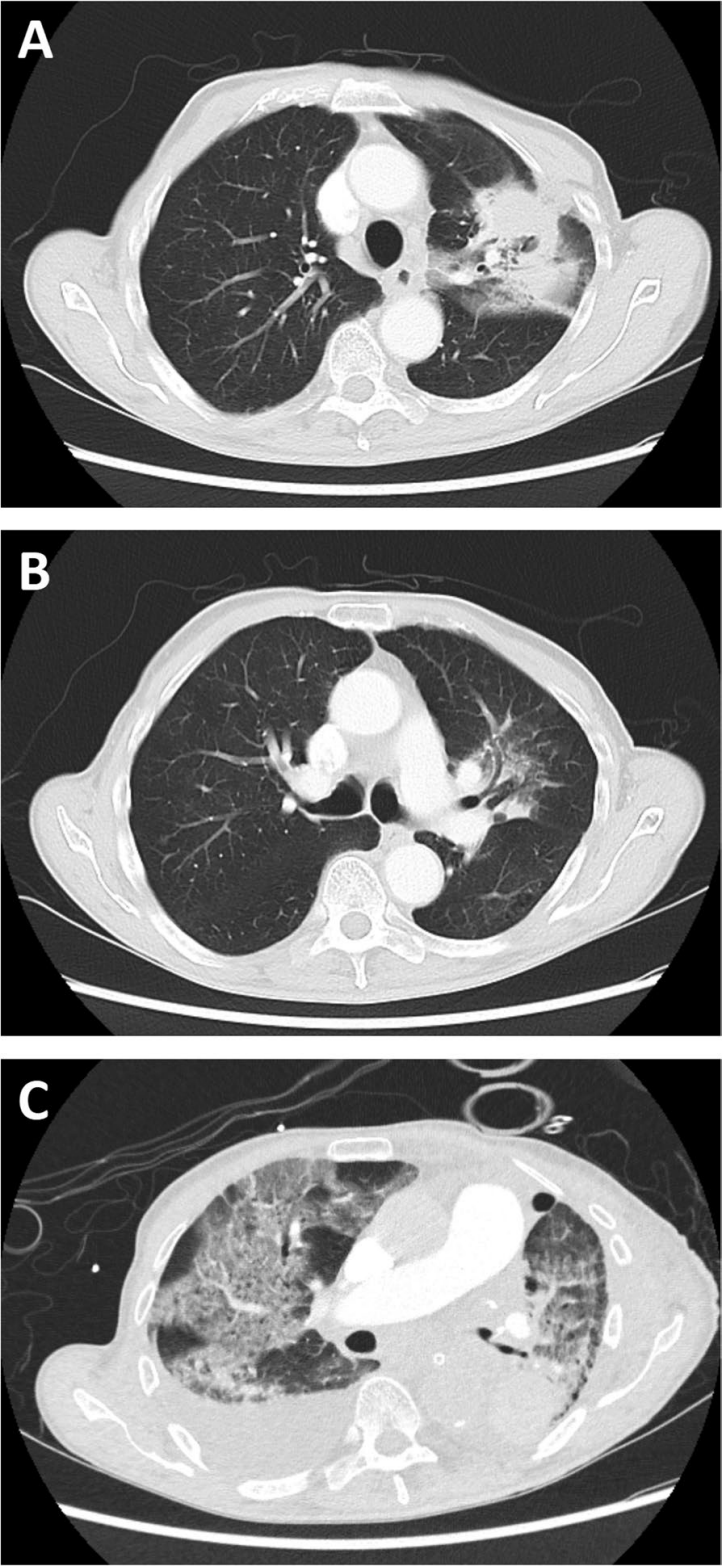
A-C Preoperative (A, B) and postoperative (C) transverse CT images of the thorax
